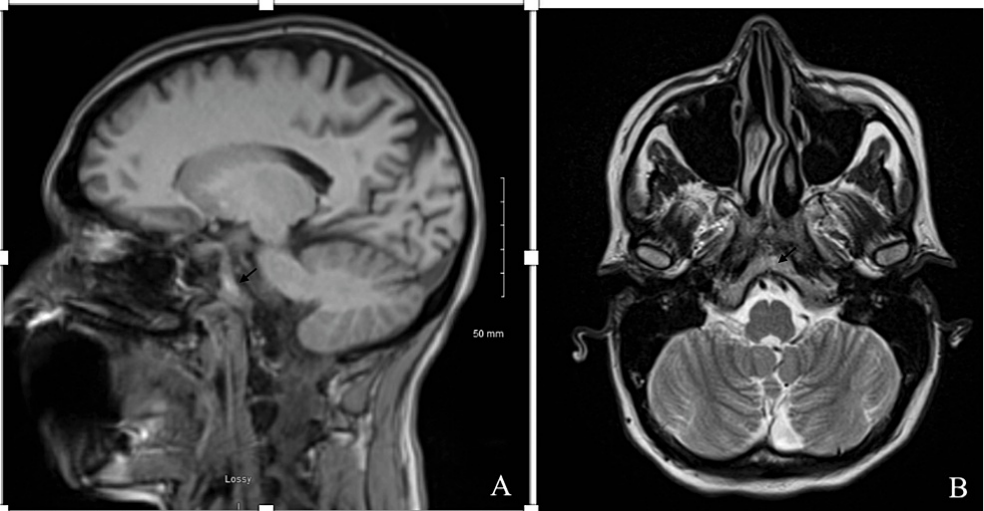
T1-weighted MRI of the brain showing subarachnoid hemorrhage within the basal cisterns in sagittal (A) and axial (B) planes.
